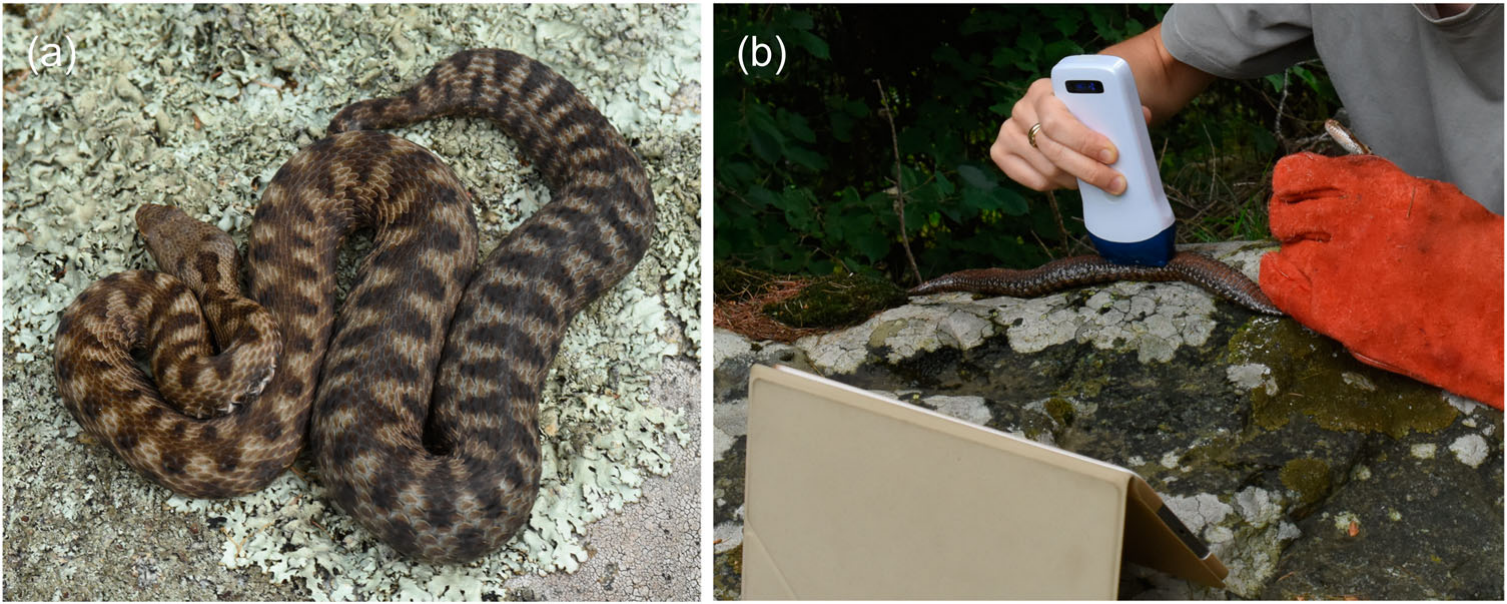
Ultrasound examination of vipers (*Vipera aspis*). (a) One of the gravid females that were investigated. (b) In‐the‐field ultrasound scanning of a gravid viper. Females were examined shortly after capture and then released at the exact capture site.
